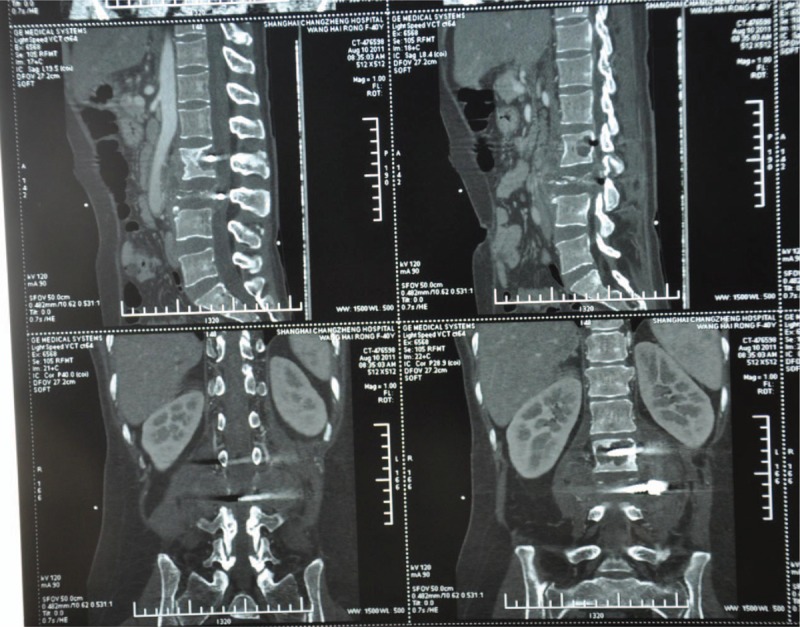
Preoperative CT images of the patient showed that osseous destruction in L2, obvious compression of centrum in L3. CT = computed tomography.
